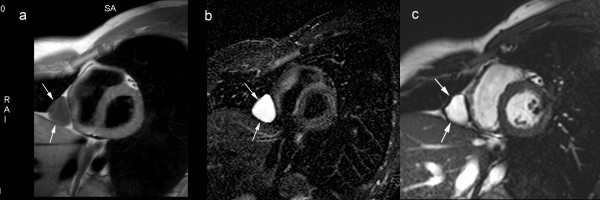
Congenital pericardial cyst. T1-weighted spin-echo CMR. (a) T2-weighted short-tau inversion-recovery spin-echo CMR (b), and cine CMR (c) in cardiac short-axis. The pericardial cyst is visible as a well-delineated, homogeneous soft-tissue structure (arrows) along the right paracardiac border implanted on a normal pericardium. The cyst content typically has signal characteristics of water on CMR.
